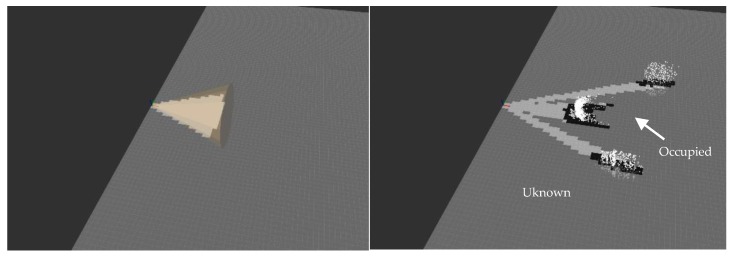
Ultrasound (left) and depth camera (right) measurements together with their respective occupancy grid computed with SigmaFusion™. The color of each cell encodes the occupancy probability (black: “occupied”; light grey: “empty”, grey: “unknown”).
